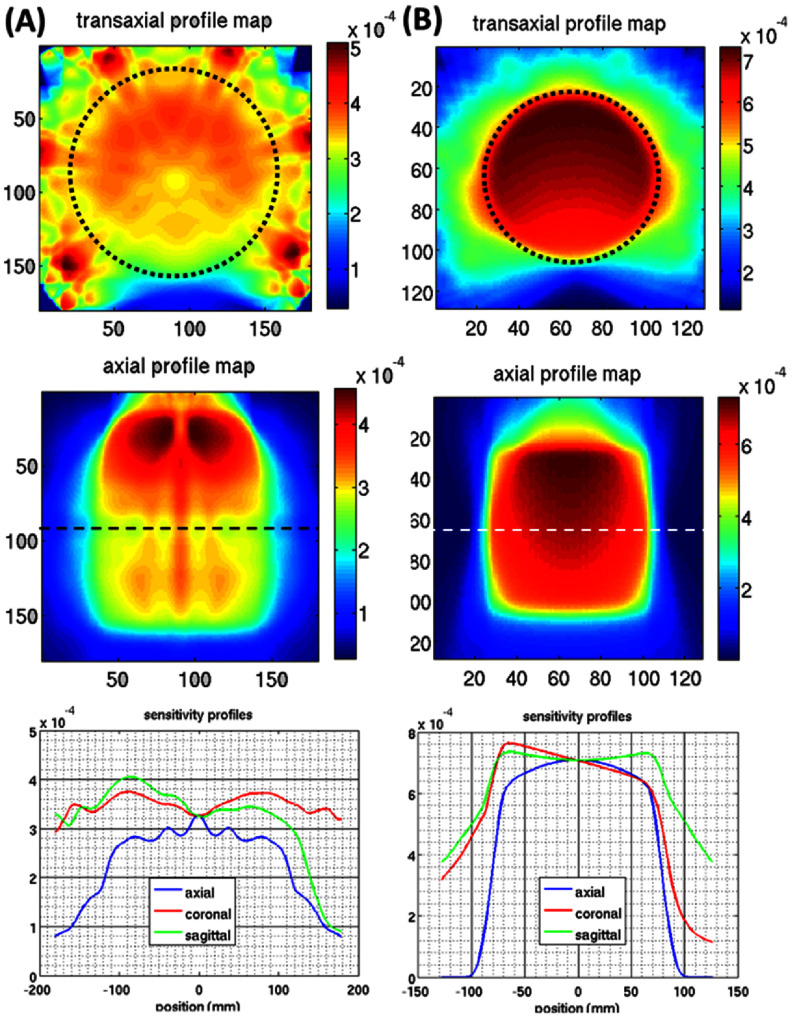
The DE-SPECT system sensitivity: (A) wide-FOV imaging configuration and (B) HR-HS FOV imaging configuration. (1st row) Trans-axial and (2nd row) axial sensitivity maps, (3rd row) sensitivity profiles. The circled dotted lines show the transaxial FOV, and the dashed lines show the gantry axis.
